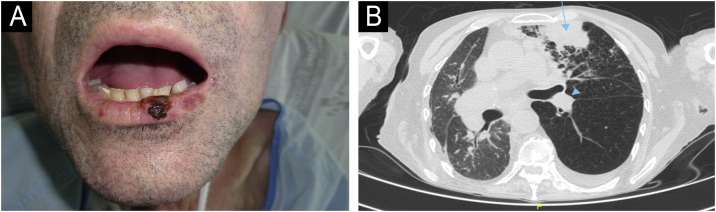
Patient Number 1. In Patient 1, a cutaneous squamous cell carcinoma (cSCC) measuring 1.3 × 1 cm is observed on the lower lip (A). The axial computed tomography (CT) scan (B) reveals a mass consistent with pulmonary metastasis in the anterior segment of the upper left lobe (blue arrow), along with an associated left paratracheal metastasis (blue arrowheads).
